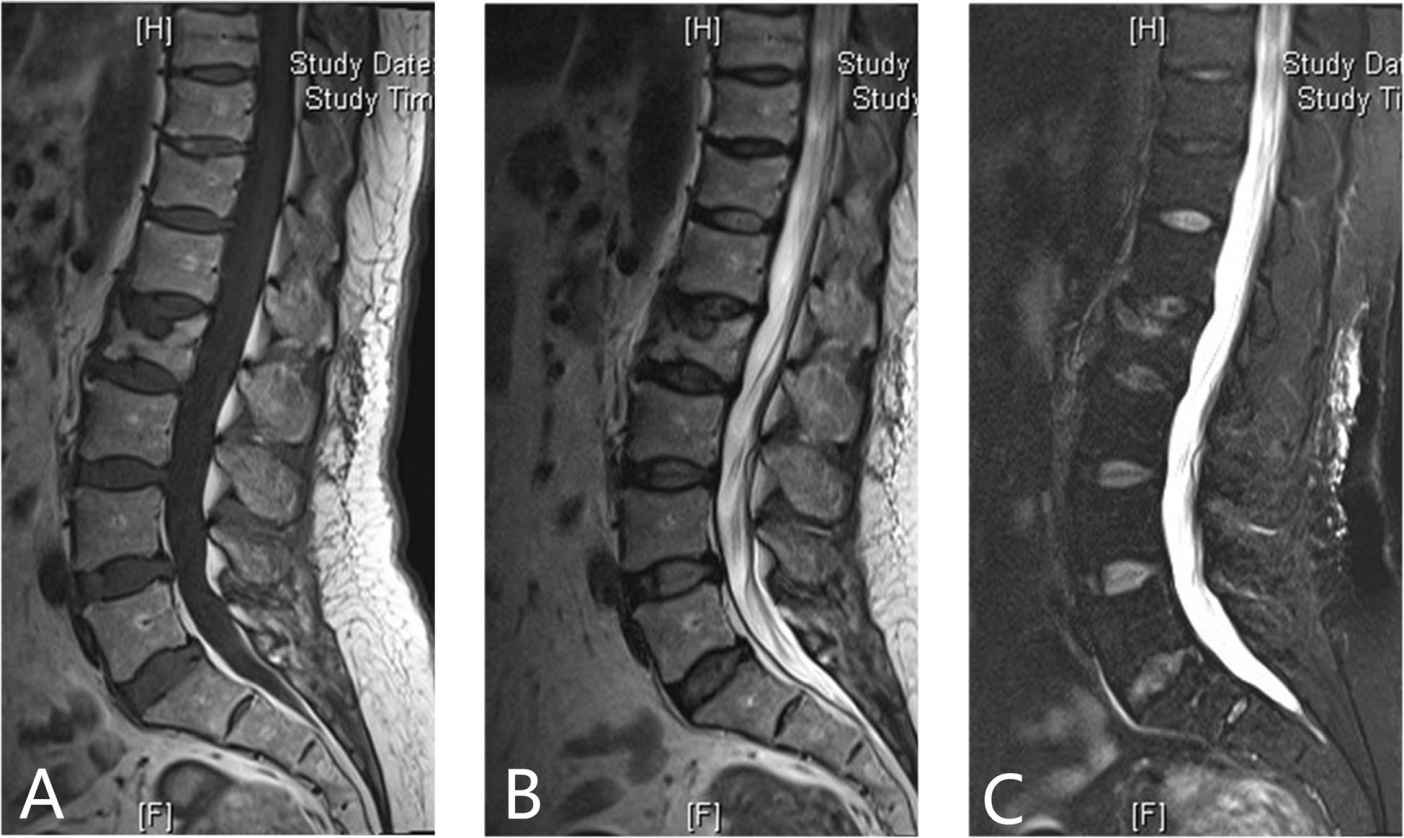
Pre-operation sagittal lumbar MRI demonstrates a large SN at the inferior endplate of L2 on T1WI and T2WI sequences (a, b), and high signal intensity in T2 inferior endplate around SN on fat-suppression sequences (c)
